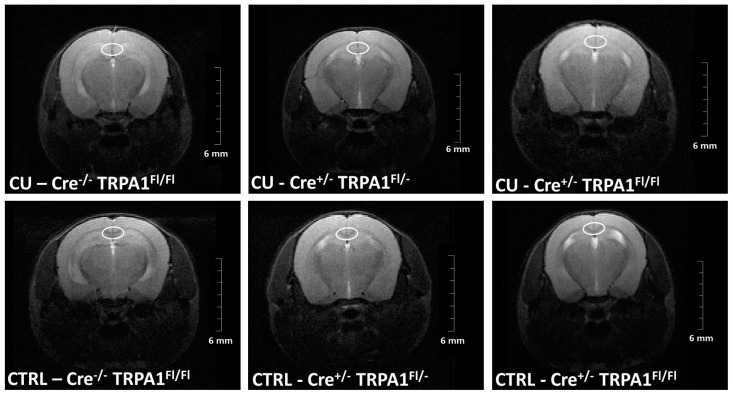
Representative MR images, constructed on fourth week. The medial corpus callosum is highlighted by a white ellipse.
